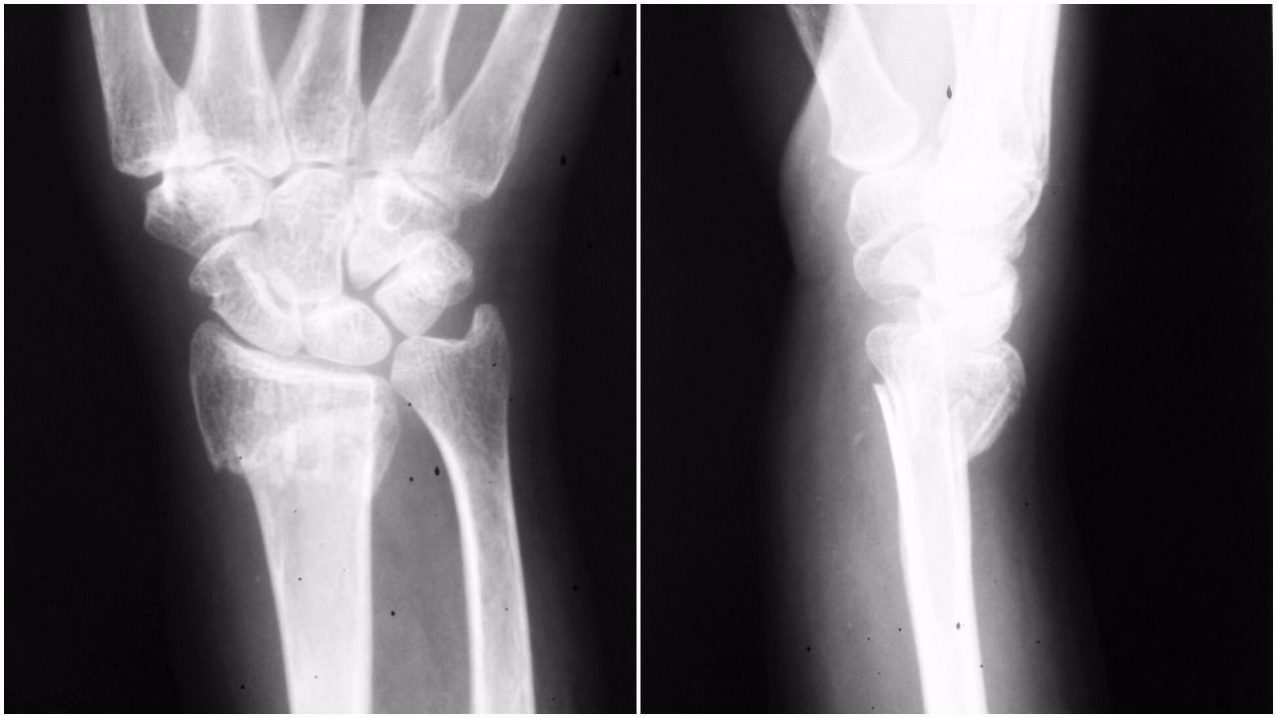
Three-month postoperative x-ray of left distal radius - anteroposterior and lateral view.
